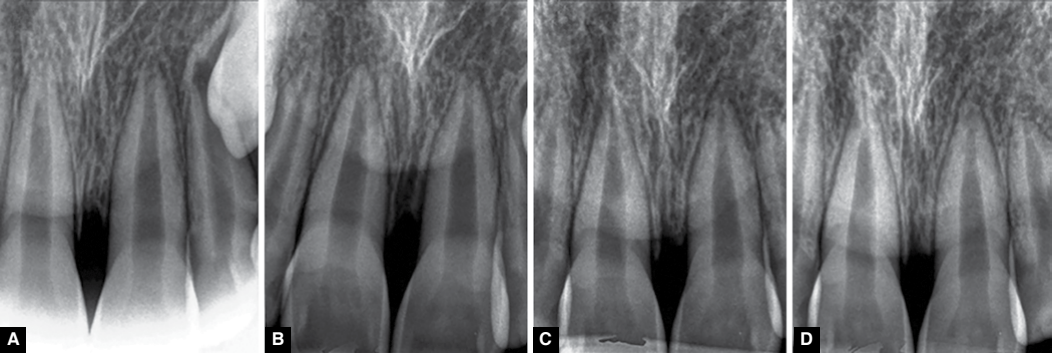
Intraoral periapical digital radiograph (A): At 3 months (B): At 6 months (C): At 12 months (D): At 18 months
